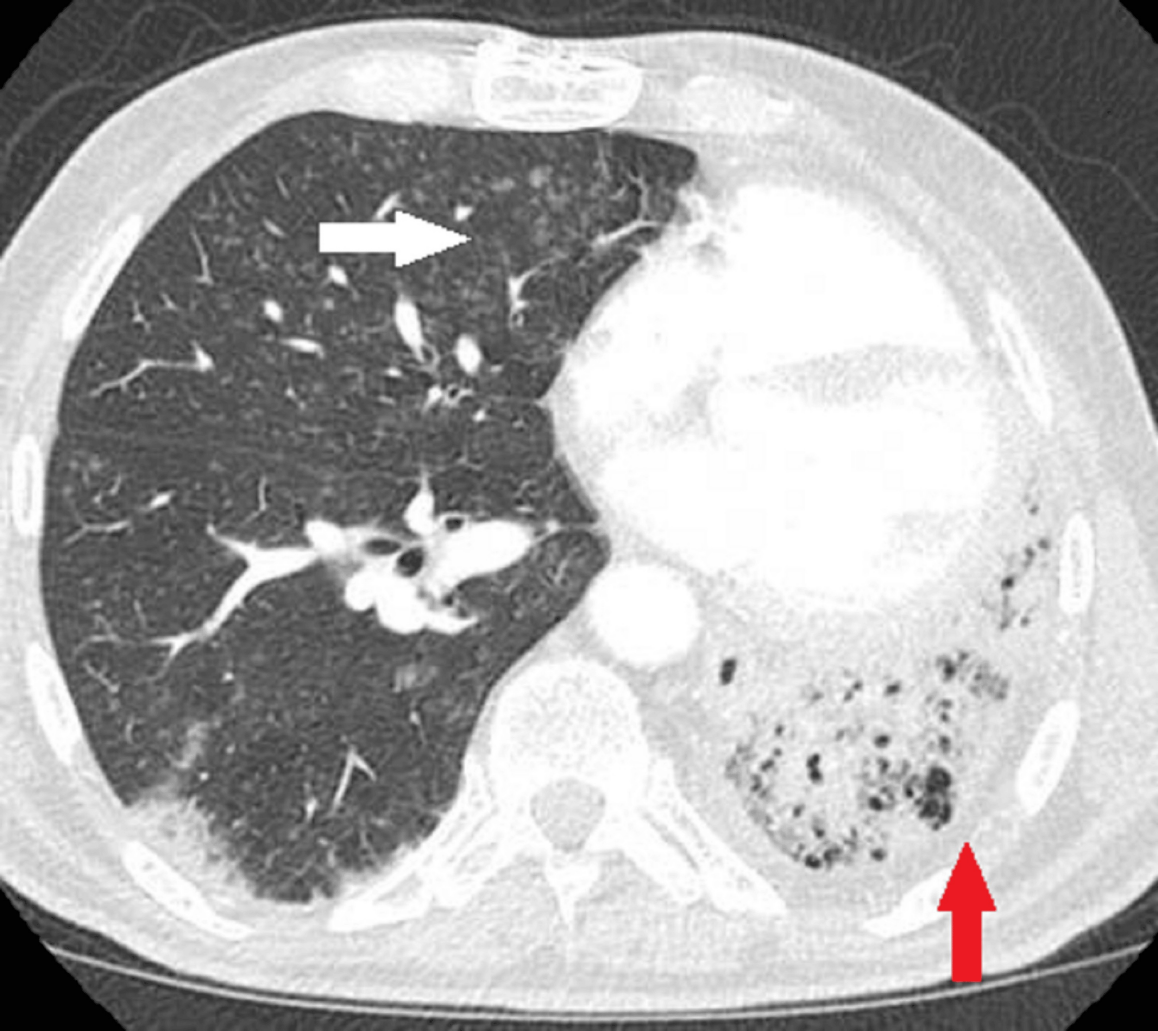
Lung window, axial view. The CT image demonstrates decreased left lung volume relative to the right with mediastinal shift to the left. There is extensive left lung airspace consolidation with honeycombing in the left lower lobe (red arrow), as well as bronchiectasis, consistent with asymmetric pulmonary fibrosis of the left lung. On the contralateral lung (right), there are ground-glass nodules in the right middle and right lower lobes (white arrow) with small volume consolidation in the dependent right lower lobe.
